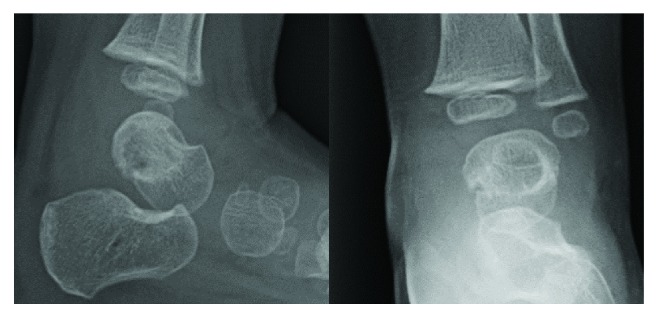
Lateral and AP plain films of the postoperative ankle.
